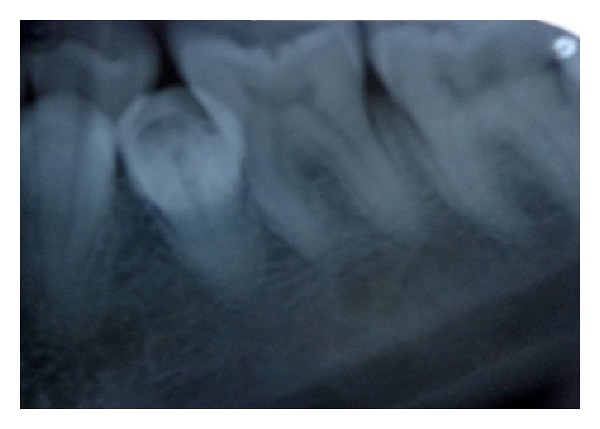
Intraoral periapical radiograph of the supernumerary premolars.
